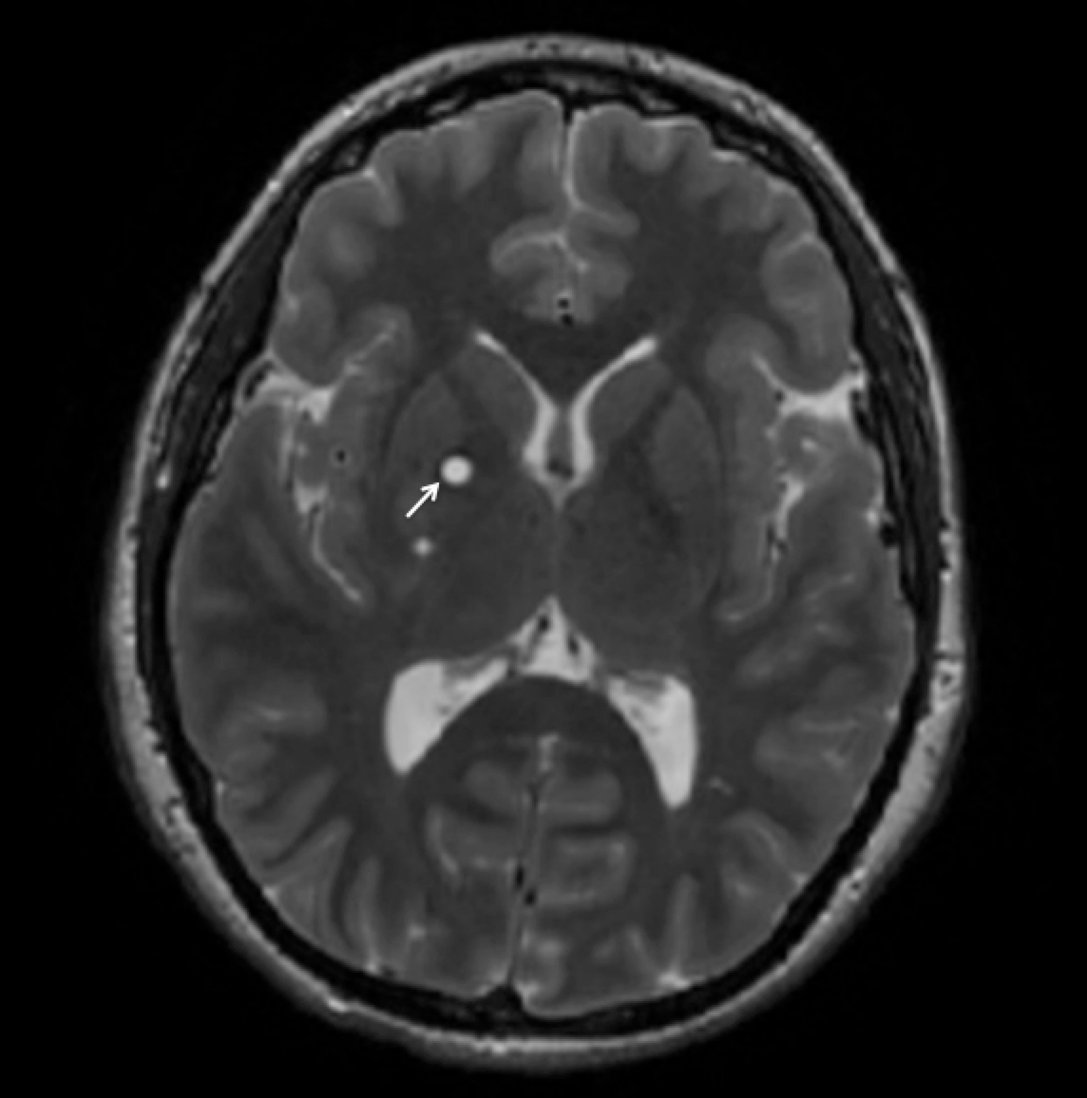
Axial T2 FLAIR MRI Hyperintense lesions within the right lentiform nucleus concerning for glioma.
